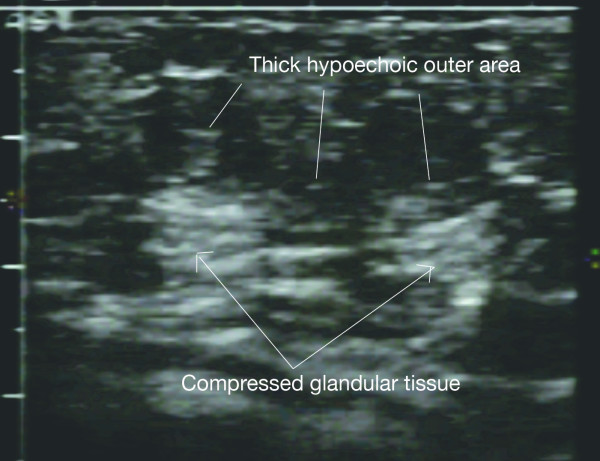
Blocked duct presents as a large area of breast tissue of altered echogenicity on ultrasound. The central area is increased in echogenicity and surrounded by a more hypoechoic rim. A large wedge shaped hardened area was felt on palpation.
